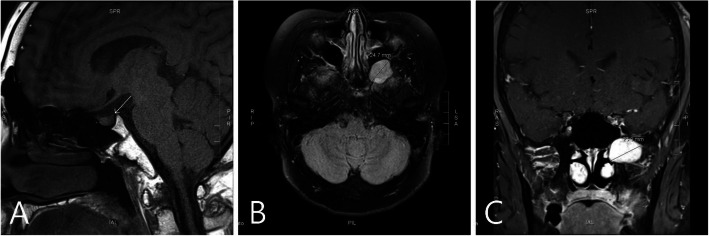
Magnetic resonance image of the sella turcica. Sagittal T1W1 (a). Axial T2W1 (b). Coronary enhanced T1W1 (c). Loss of normal T1 hyperintensity was observed in the posterior lobe of the pituitary gland (arrow). An enhanced 2.5-cm mass suspected of being a schwannoma is visible in the area of the left pterygopalatine fossa
